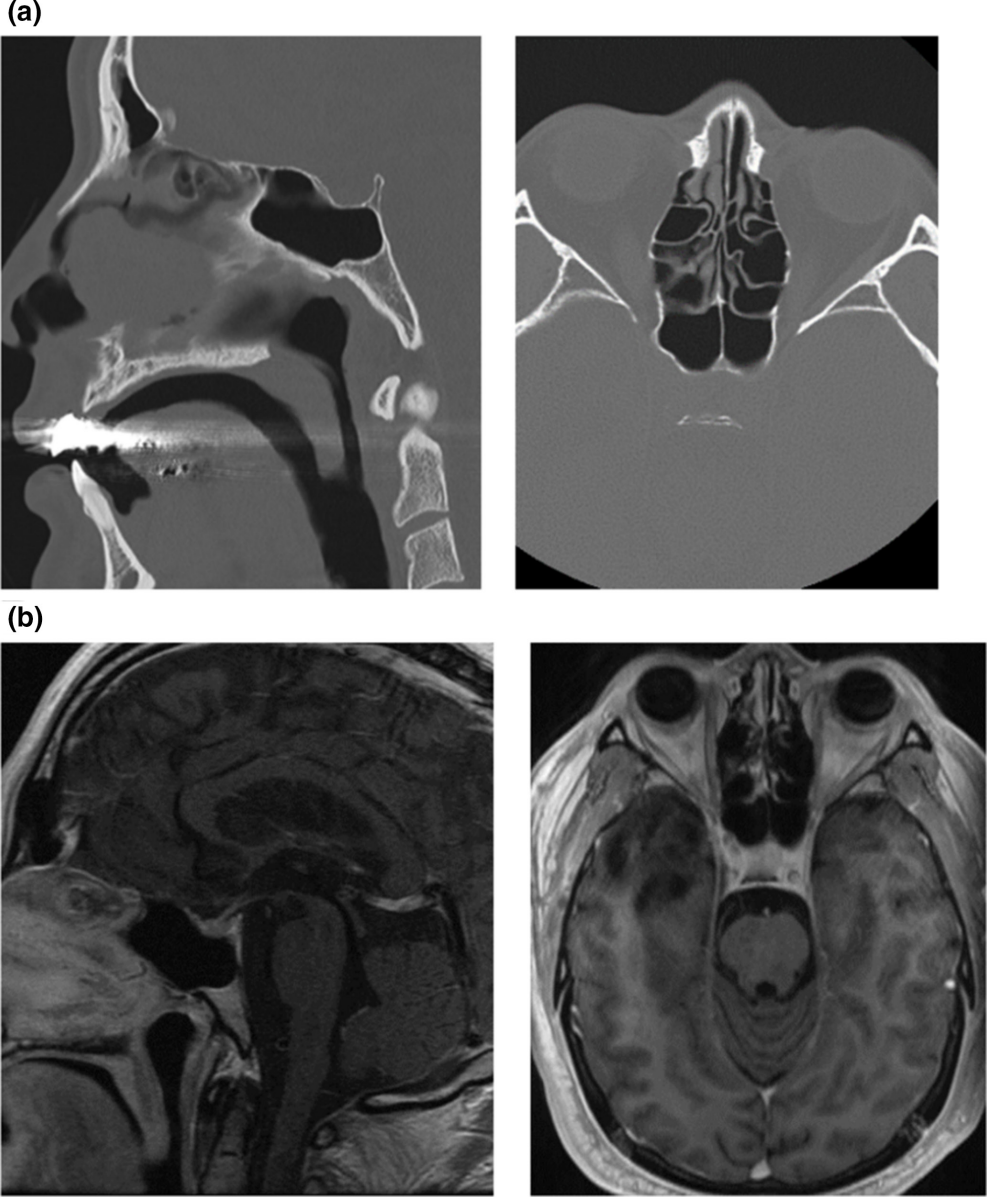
Resolution of the pituitary expansion and erosion of the sella turcica. (a) CT sinus bone windows demonstrates resolution of herniation of pituitary tissue and repair of sellar defect. (b) Post-contrast T1-weighted MRI demonstrates resolution of pituitary tissue herniation with normal enhancement pattern.
